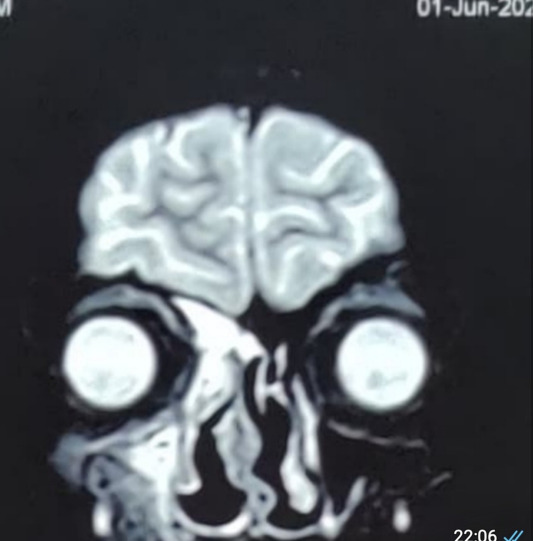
T2 weighted coronal section on MRI showing involvement of the right frontal and maxillary sinuses
